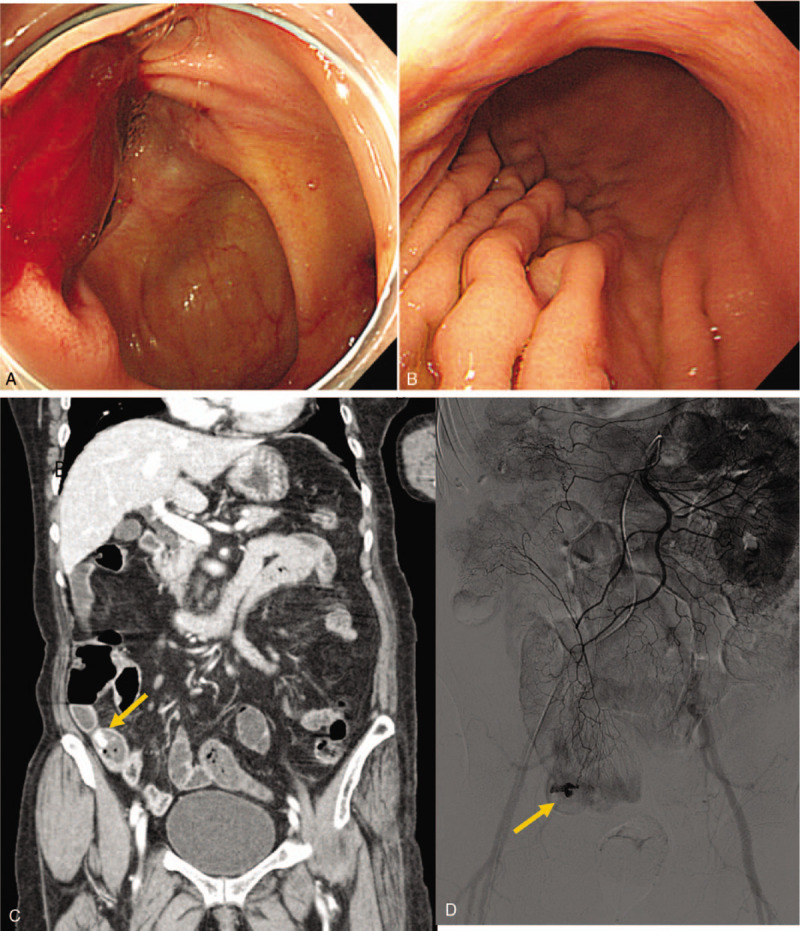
79-year-old female with hematochezia. A. initially performed colonoscopy revealing bleeding from the ileum, B. esophagogastroduodenoscopy showing non-specific finding, C. portal phase computed tomography showing active bleeding at the distal ileum with uncertain primary cause. D. angiography confirming the active bleeding. E. selective glue embolization was subsequently performed.
